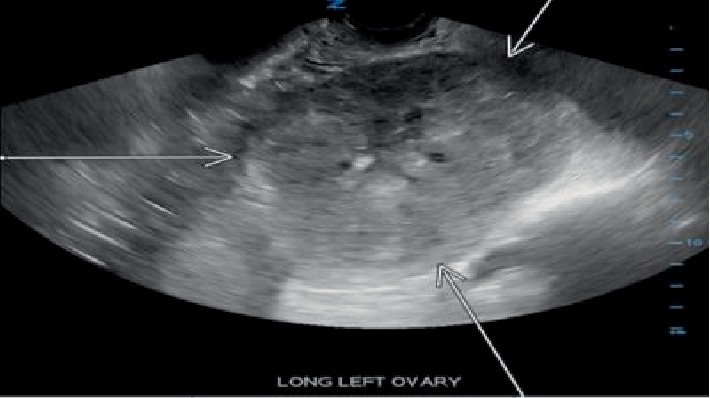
Ultrasound showed a solid hypervascular, mostly hypoechoic, partially isoechoic/partially hypoechoic left ovarian mass measuring 12.6 × 9.8 × 9.4 cm (outline by arrows).
